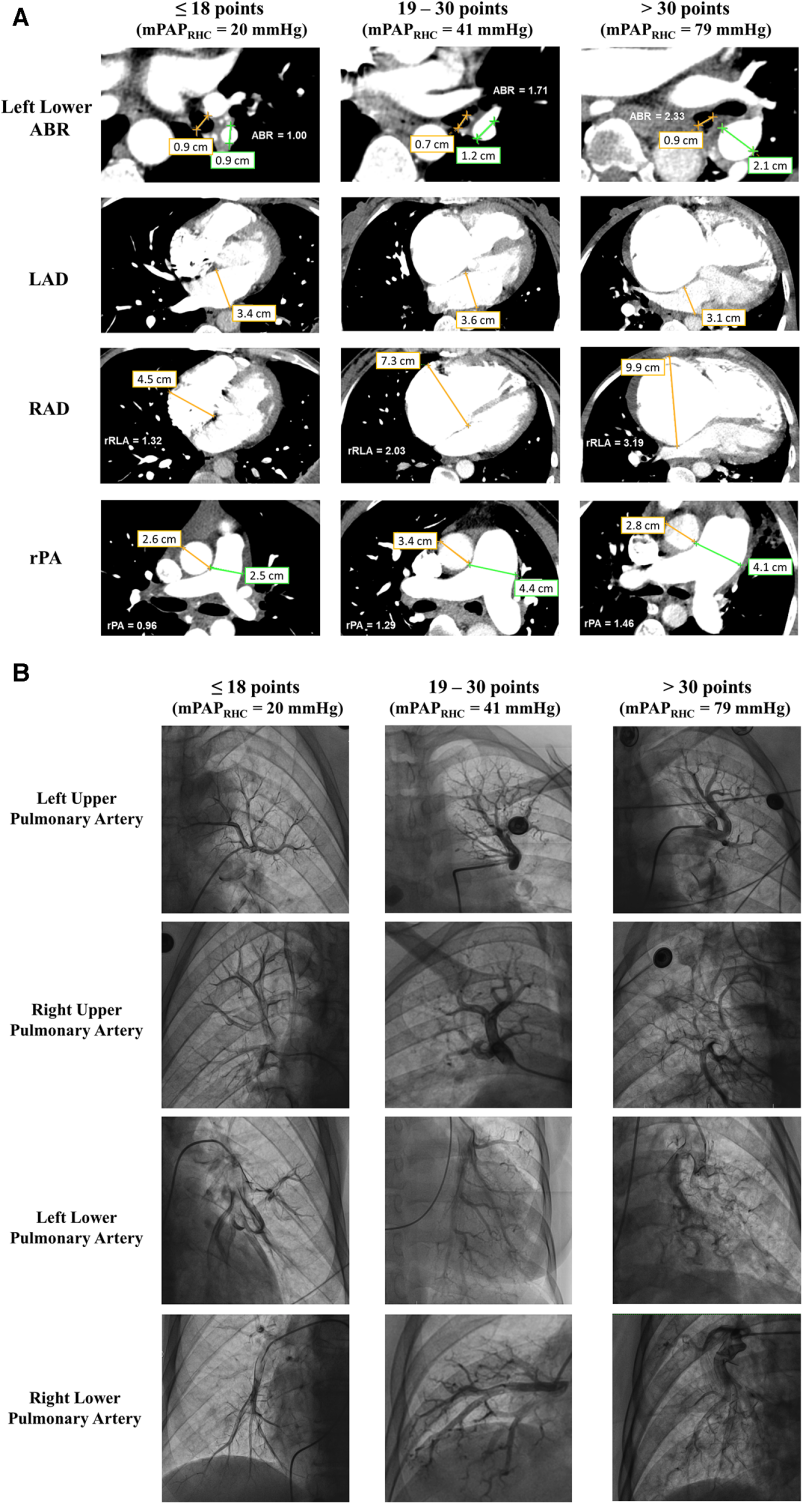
Imaging features of CTA and pulmonary arteriography in the GENTH score. (A) The diameter of the left lower pulmonary artery and bronchus, right and left atria, aorta, and main pulmonary artery in patients with different GENTH scores in the validation set. (B) Imaging features of pulmonary arteriography in patients with different GENTH scores in the validation set. ABR, pulmonary artery-bronchus ratio; LAD, left atrium diameter; RAD, right atrium diameter; rPA, ratio of the main pulmonary artery to aorta diameter; rRLA, ratio of the right to left atrial diameter.
